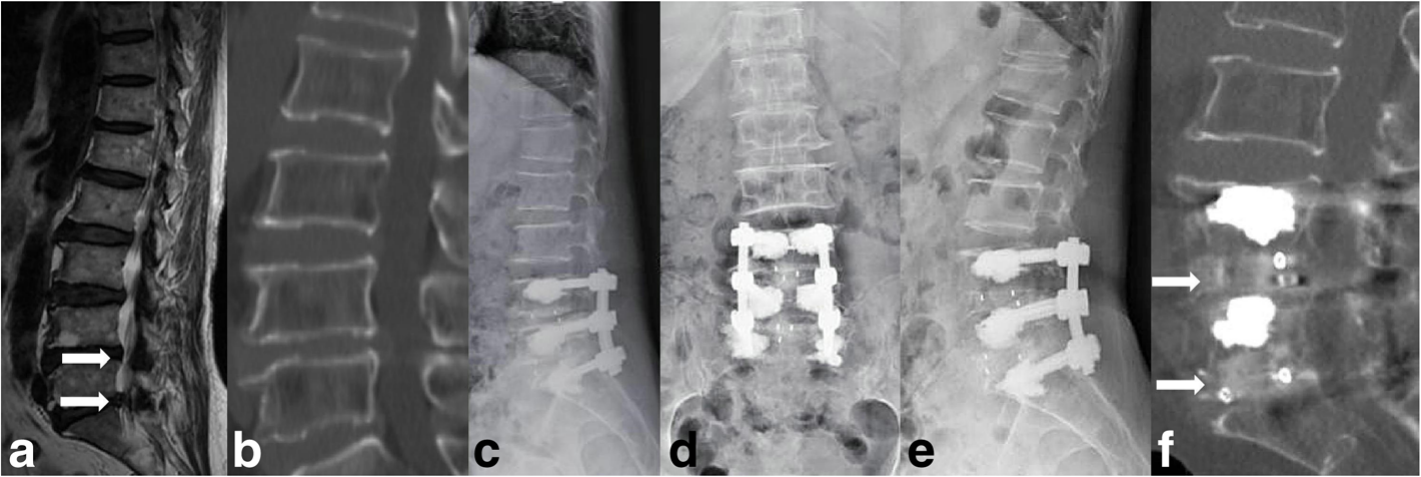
Radiological images of a representative case with polymethylmethacrylate-augmented pedicle screw treatment. a–f A 67-year-old female with lumbar spinal stenosis in L4/5 and L5/S1 segments. a Preoperative MRI image showed obvious spinal stenosis in L4/5 and L5/S1 segments (white arrow). b Preoperative CT scanning showed no osteophyte in posterior of vertebrae. c–d Postoperative X-ray showed the normal position of pedicle screws and the cement in the vertebrae. e Lateral X-ray at 36 months after fusion surgery showed normal position of pedicle screws. f Lumber CT scanning at 36 months after fusion surgery showed series cancellous bone through L4/5 and L5/S1 intervertebral space (white arrow)
